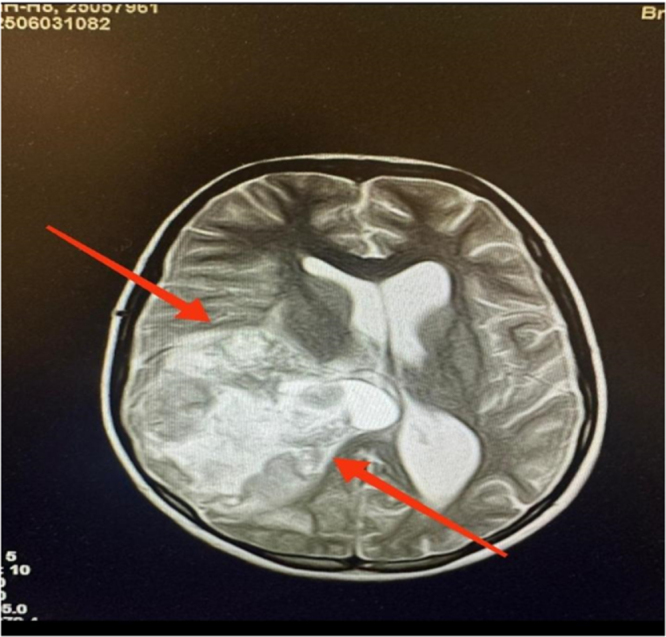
Preoperative contrast-enhanced T1-weighted MRI showing a large, lobulated, intensely enhancing mass in the right frontoparietal region with surrounding vasogenic edema and mass effect.
